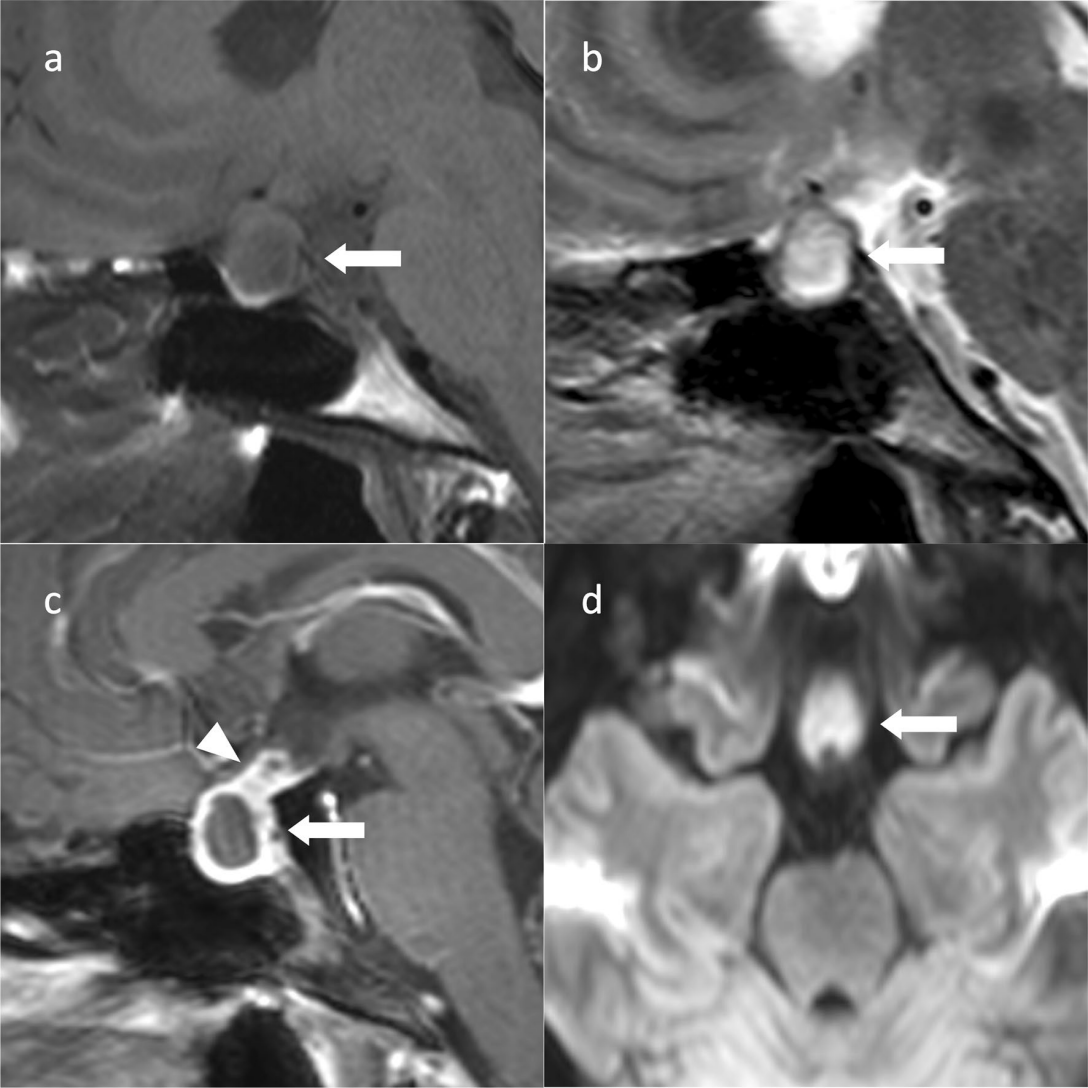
Pituitary abscess. An approximately 20-year-old female with headaches and nausea. Sagittal T1WI (a) and sagittal T2WI (b) show a cystic mass within the sellar turcica to the suprasellar region (arrow). Sagittal T2WI (b) failed to show hypointense regions of the peripituitary region (parasellar T2-dark sign). c Sagittal contrast-enhanced T1WI shows contrast enhancement of the cyst wall (arrow) with an enlarged pituitary stalk (arrowhead). d Diffusion-weighted imaging (DWI) shows marked hyperintensity inside (mean apparent diffusion coefficient = 0.5 × 10^–3^ mm^2^/s) (arrow)
